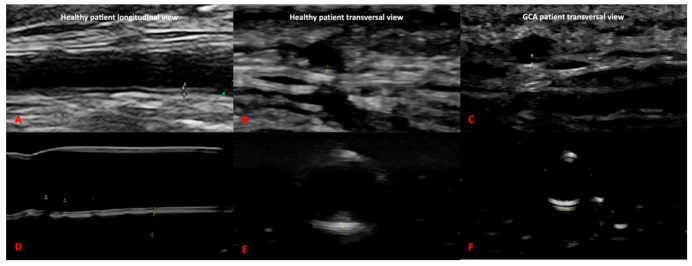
Comparison between ultrasound images of the temporal artery of the 3D printed models of healthy (D,E) and pathological setting (F) and real ultrasound images in healthy (A,B) and giant cell arteritis patients (C).
